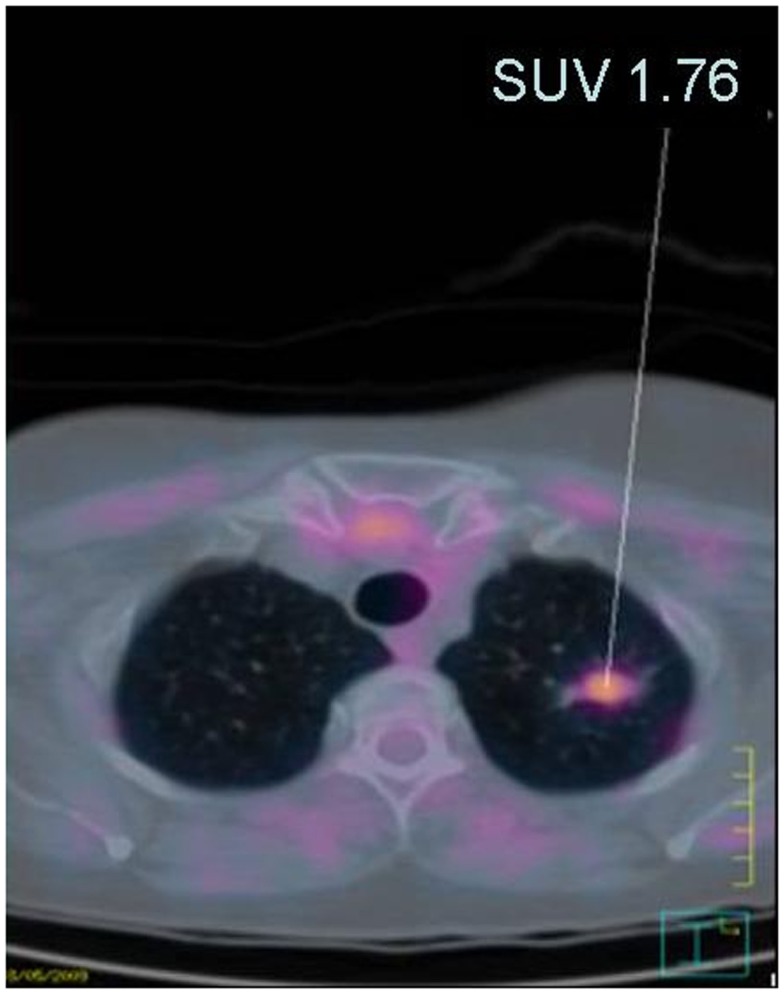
Image fusion of PET with CT showing anomalous concentration of ^18^F-FDG. A lesion localized in the apical pulmonary region is shown (SUV 1.76).
